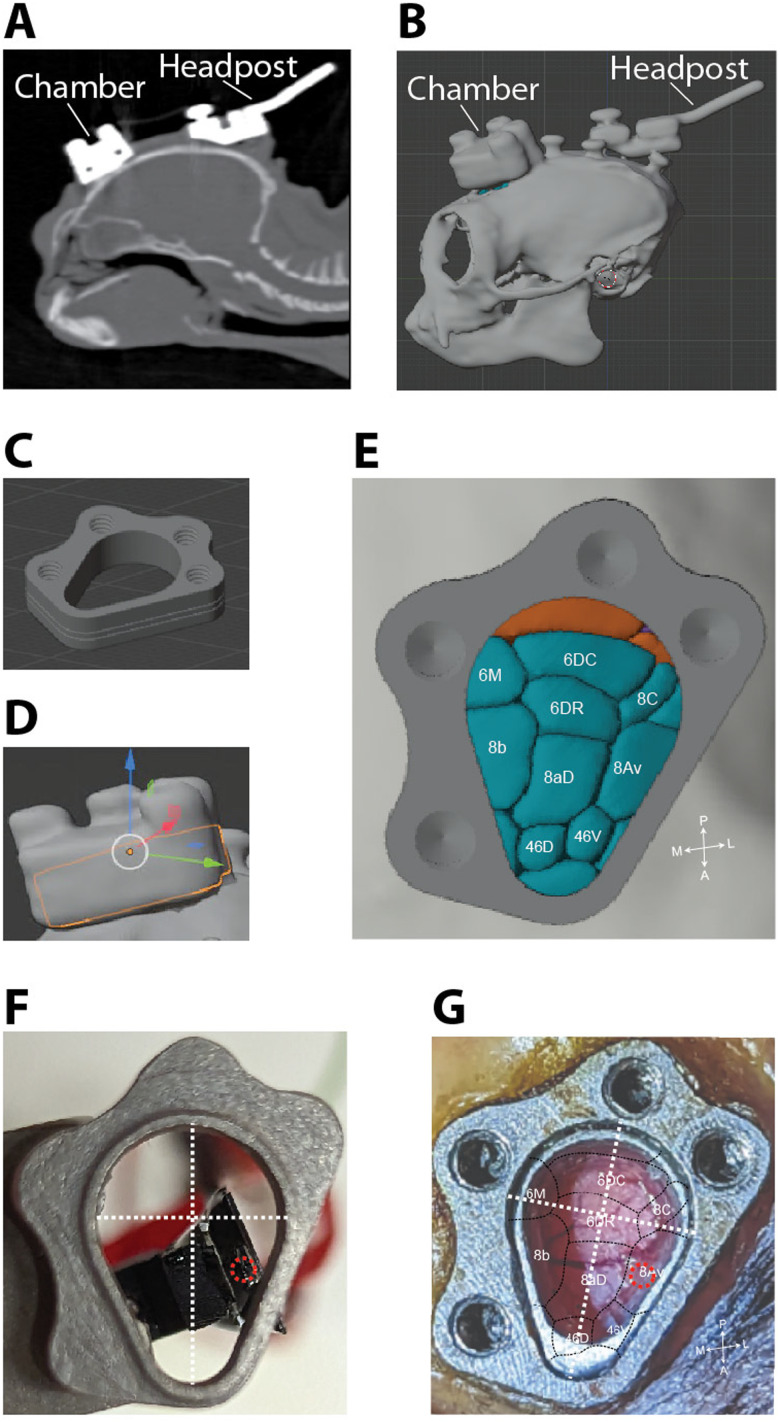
Reconstruction of recording locations. Recording locations were identified by combining 3D models and images taken of the probe and chamber. *A*, CT scan after head post and chamber implant. *B*, 3D model of skull created from CT scan. *C*, 3D model of chamber. *D*, Chamber model aligned to the chamber on the 3D model of skull. The chamber model is transparent with an orange outline. *E*, Image through chamber of 3D brain model (Liu et al., 2021; marmosetbrainmapping.org). Anterior (A), posterior (P), medial (M), and lateral (L) directions are indicated in the bottom right corner. *F*, *G*, A separate chamber is used as a reference to determine the probe location relative to the chamber. After each recording, the microdrive is mounted on a pedestal and chamber identical to the ones used for recording (*F*). A picture is taken from below and then annotated. The chamber references (white lines) and probe shank location (red circle) are placed on top of the reconstructed map of areas to identify the recording location (*G*). This particular recording session is likely in area 8aV. Double probe recording sessions are reconstructed in the same manner.
